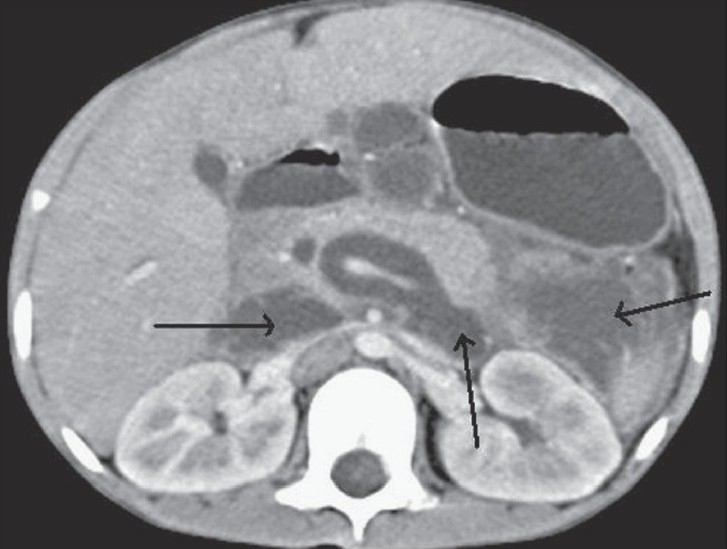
Transaxial contrast-enhanced CT scan shows a dilated intrapancreatic CBD, and a fluid collection in front of the great vessels and in the anterior left pararenal spaces (black arrows) with a pseudowall on the left
